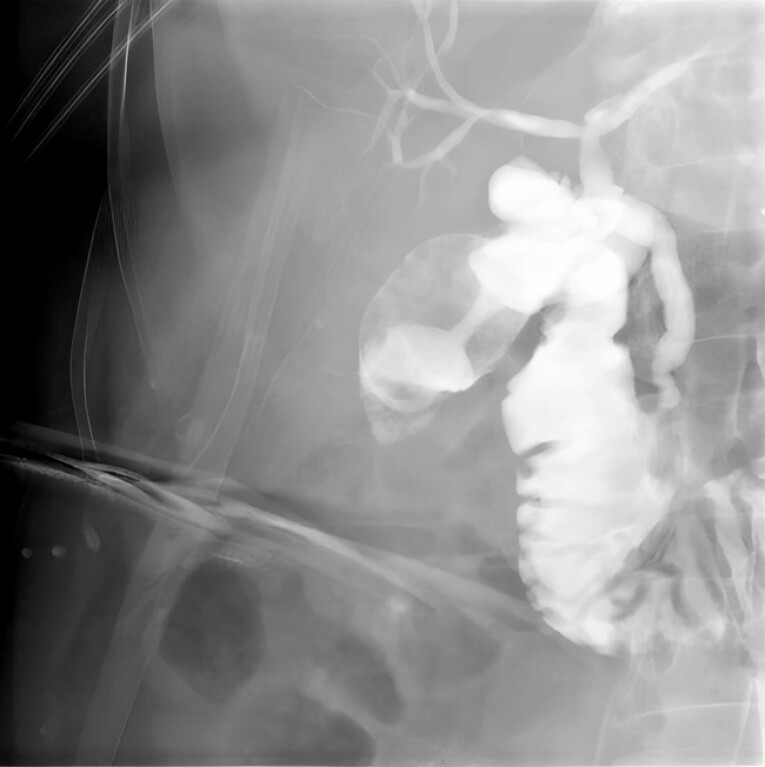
A fluoroscopic view of the first EUS-GBD, with contrast medium injection through the LAMS lumen, confirming the correct LAMS placement. EUS-GBD, endoscopic ultrasound-guided gallbladder drainage; LAMS, lumen-apposing metal stent.
